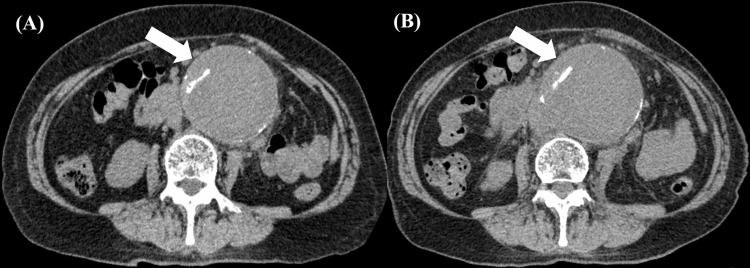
Axial abdominal CT scans A: On admission, a mycotic aneurysm of the infrarenal abdominal aorta (white arrow) was observed (size: 73 mm × 78 mm); B: On day five, the mycotic aneurysm was found to be enlarged (white arrow) despite antibiotic therapy (size: 81 mm × 85 mm)
